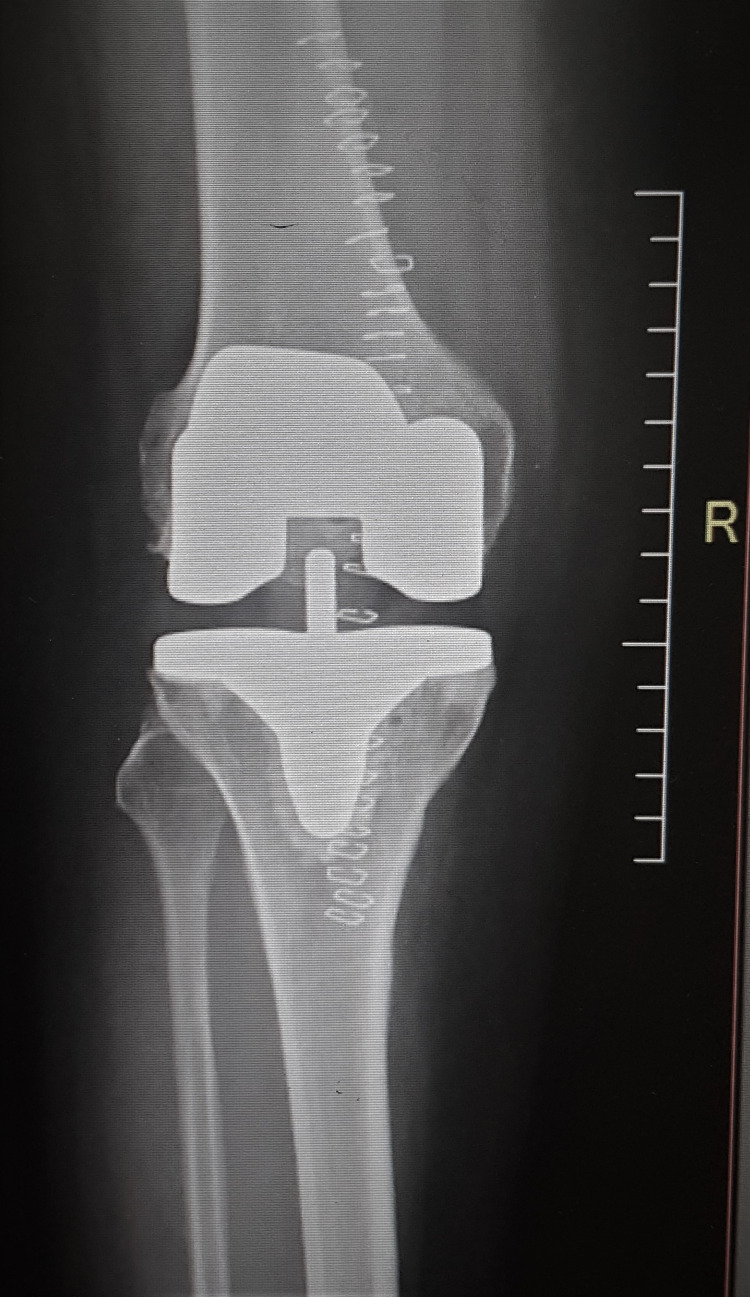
Radiograph of the right knee (anteroposterior view) with a stable implant in the immediate postoperative period
